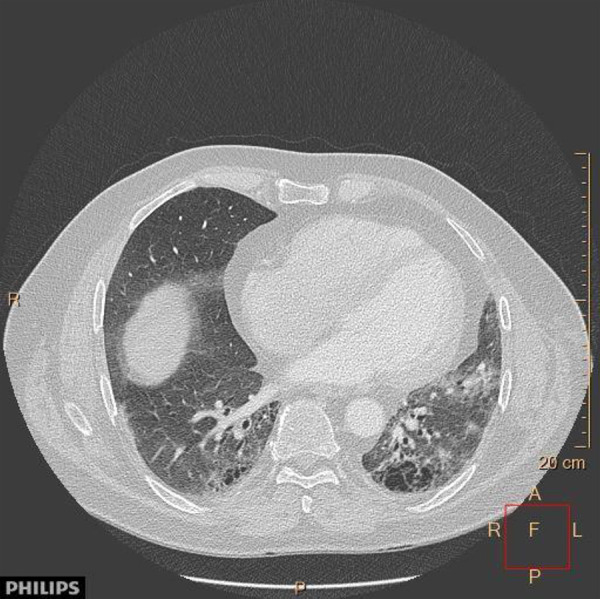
Computed tomography scan showing a florid inflammatory alveolar process with ubiquitous ground glass-like infiltrates in both lungs, cystic transformation, fibrotic changes, and septal thickening in the lower-lung zone with left-sided predominance and dilatation of the bronchi of both lower lobes.
